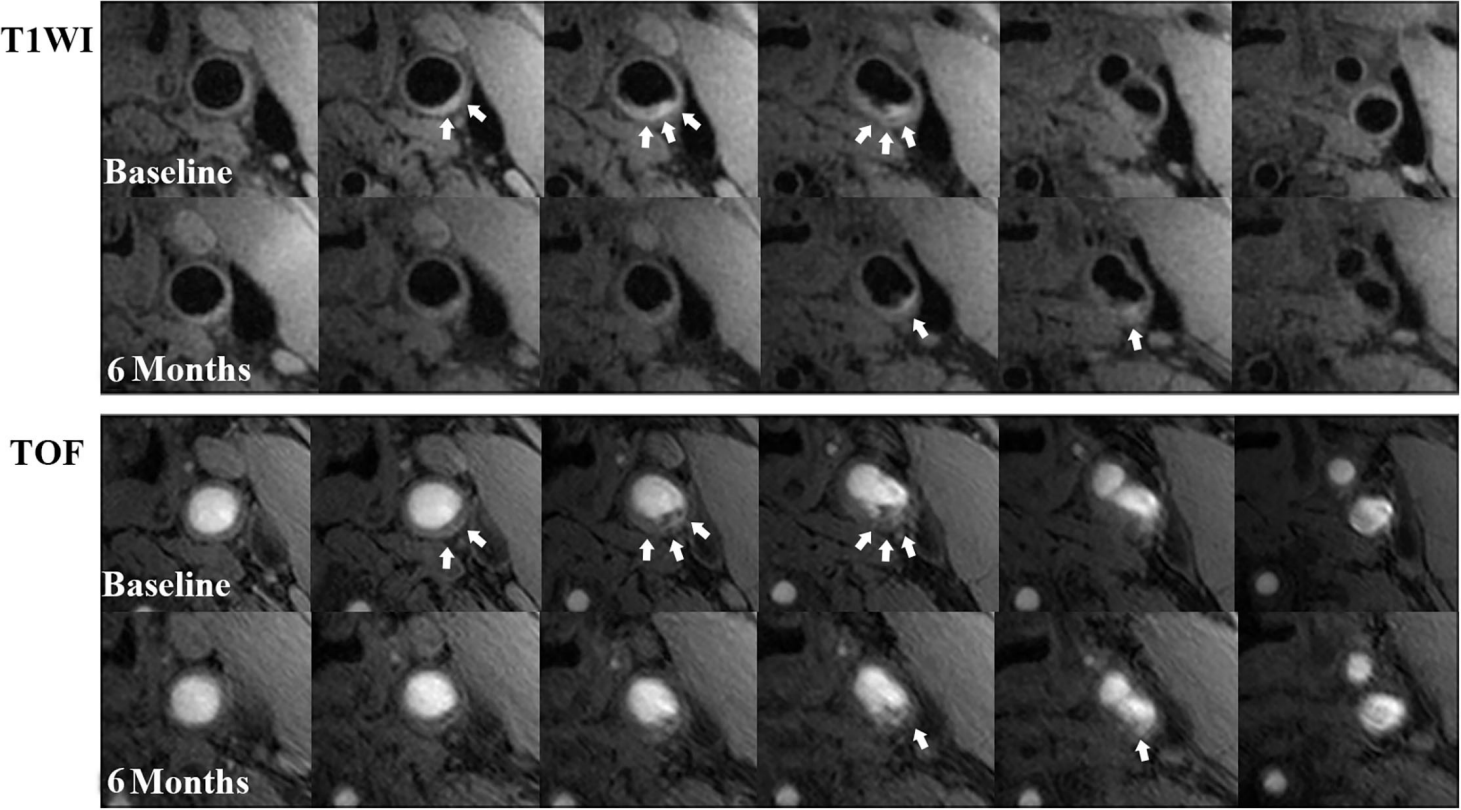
Examples of IPH regression during a 6-month follow-up. There is a remarkable regression in IPH volume (white arrows) at left carotid common artery with atherosclerosis on T1W (above 2 rows) and TOF (below 2 rows) images from the baseline to the follow-up. On some slices of the follow-up MR images, the hyperintense signal (IPH) disappeared compared with those at baseline.
